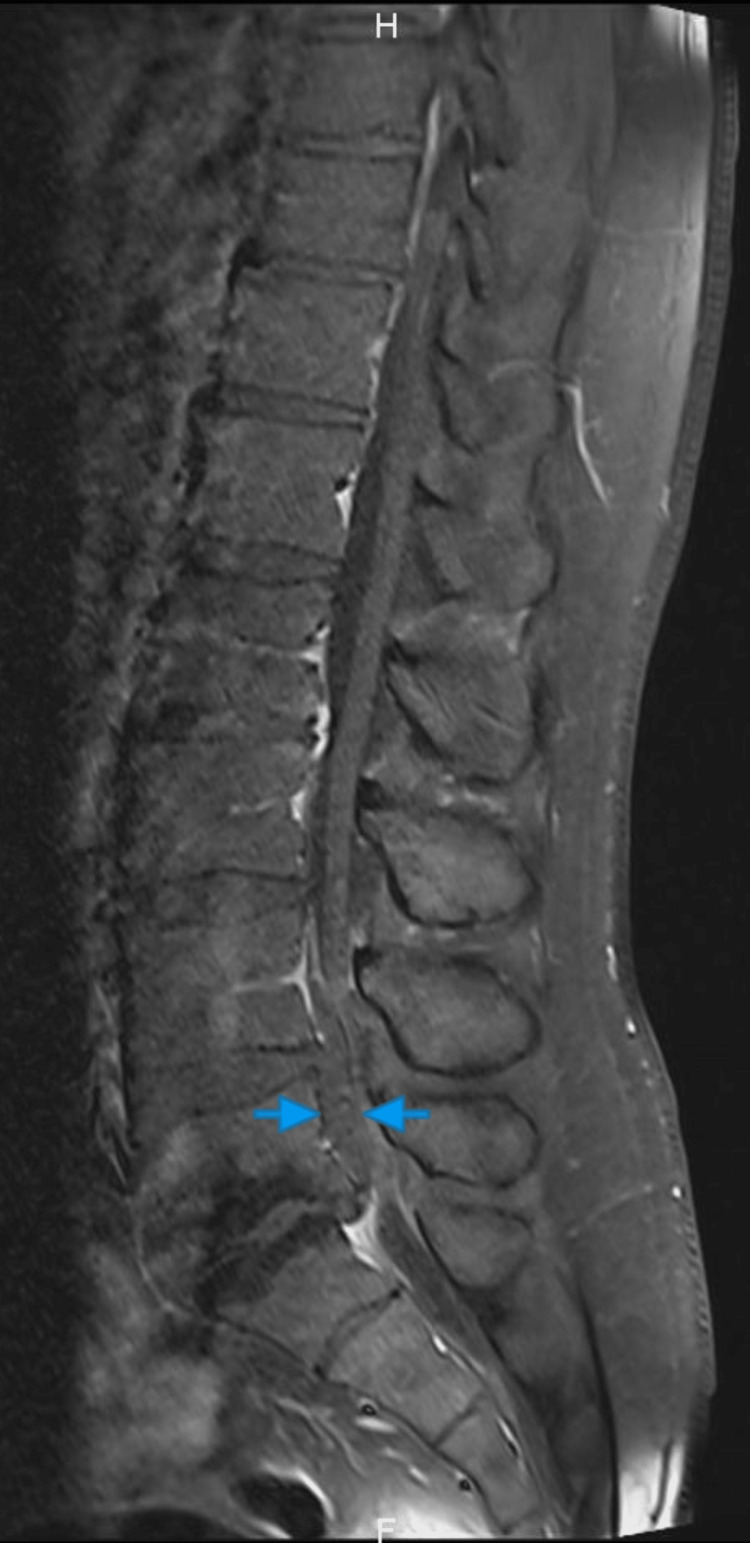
T1-weighted sagittal cut with gadolinium injection. No enhancement is observed in or around the mass, ruling out epiduritis. The mass is indicated by blue arrows.
